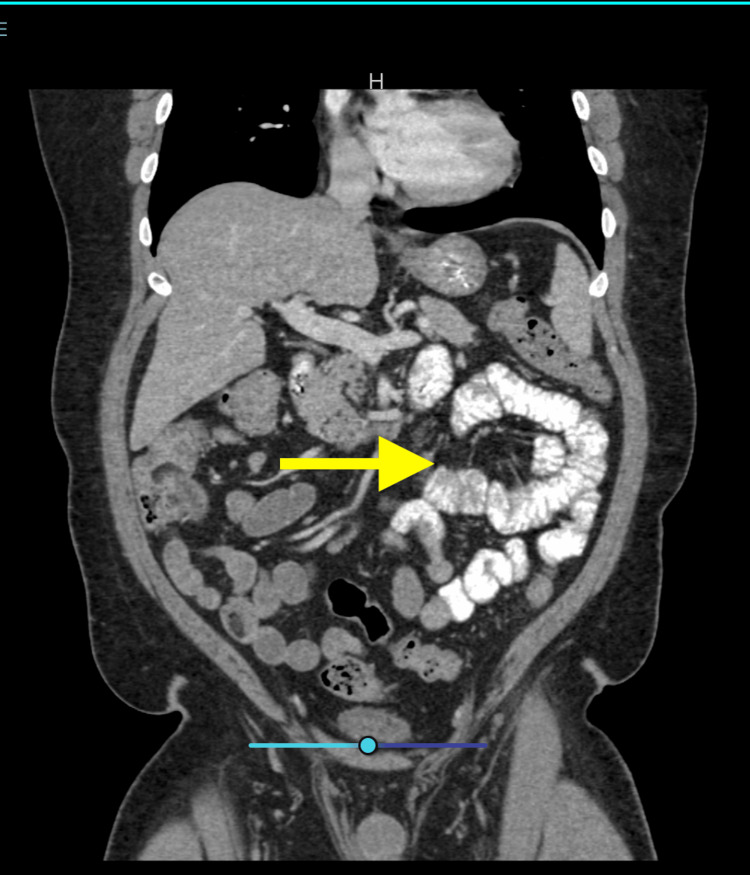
Computed tomography of the abdomen from the referring hospital demonstrated mural thickening and mucosal hyperenhancement of the terminal ileum and right colon with mild surrounding fat stranding, findings consistent with infectious enterocolitis.
